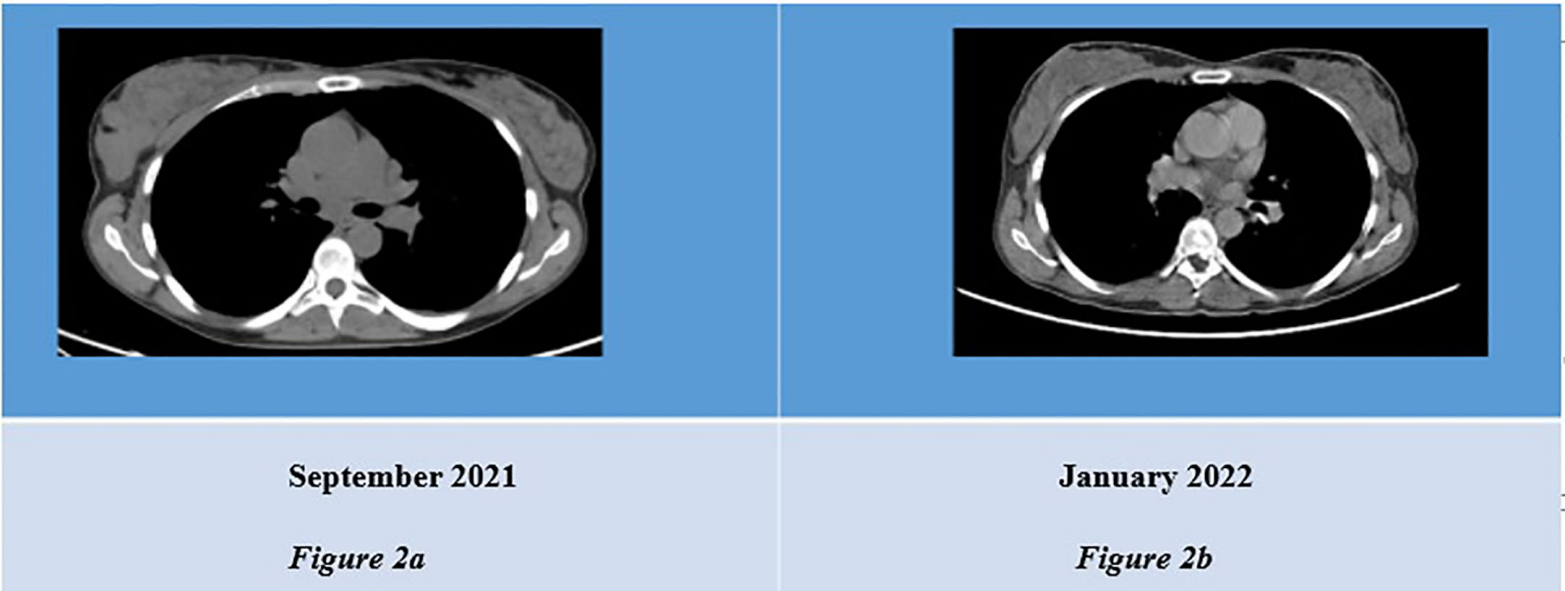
(A) CT scan showing thickened right mammal gland. (B) Revaluation CT scan showing reduced mass in infero-external right breast.
